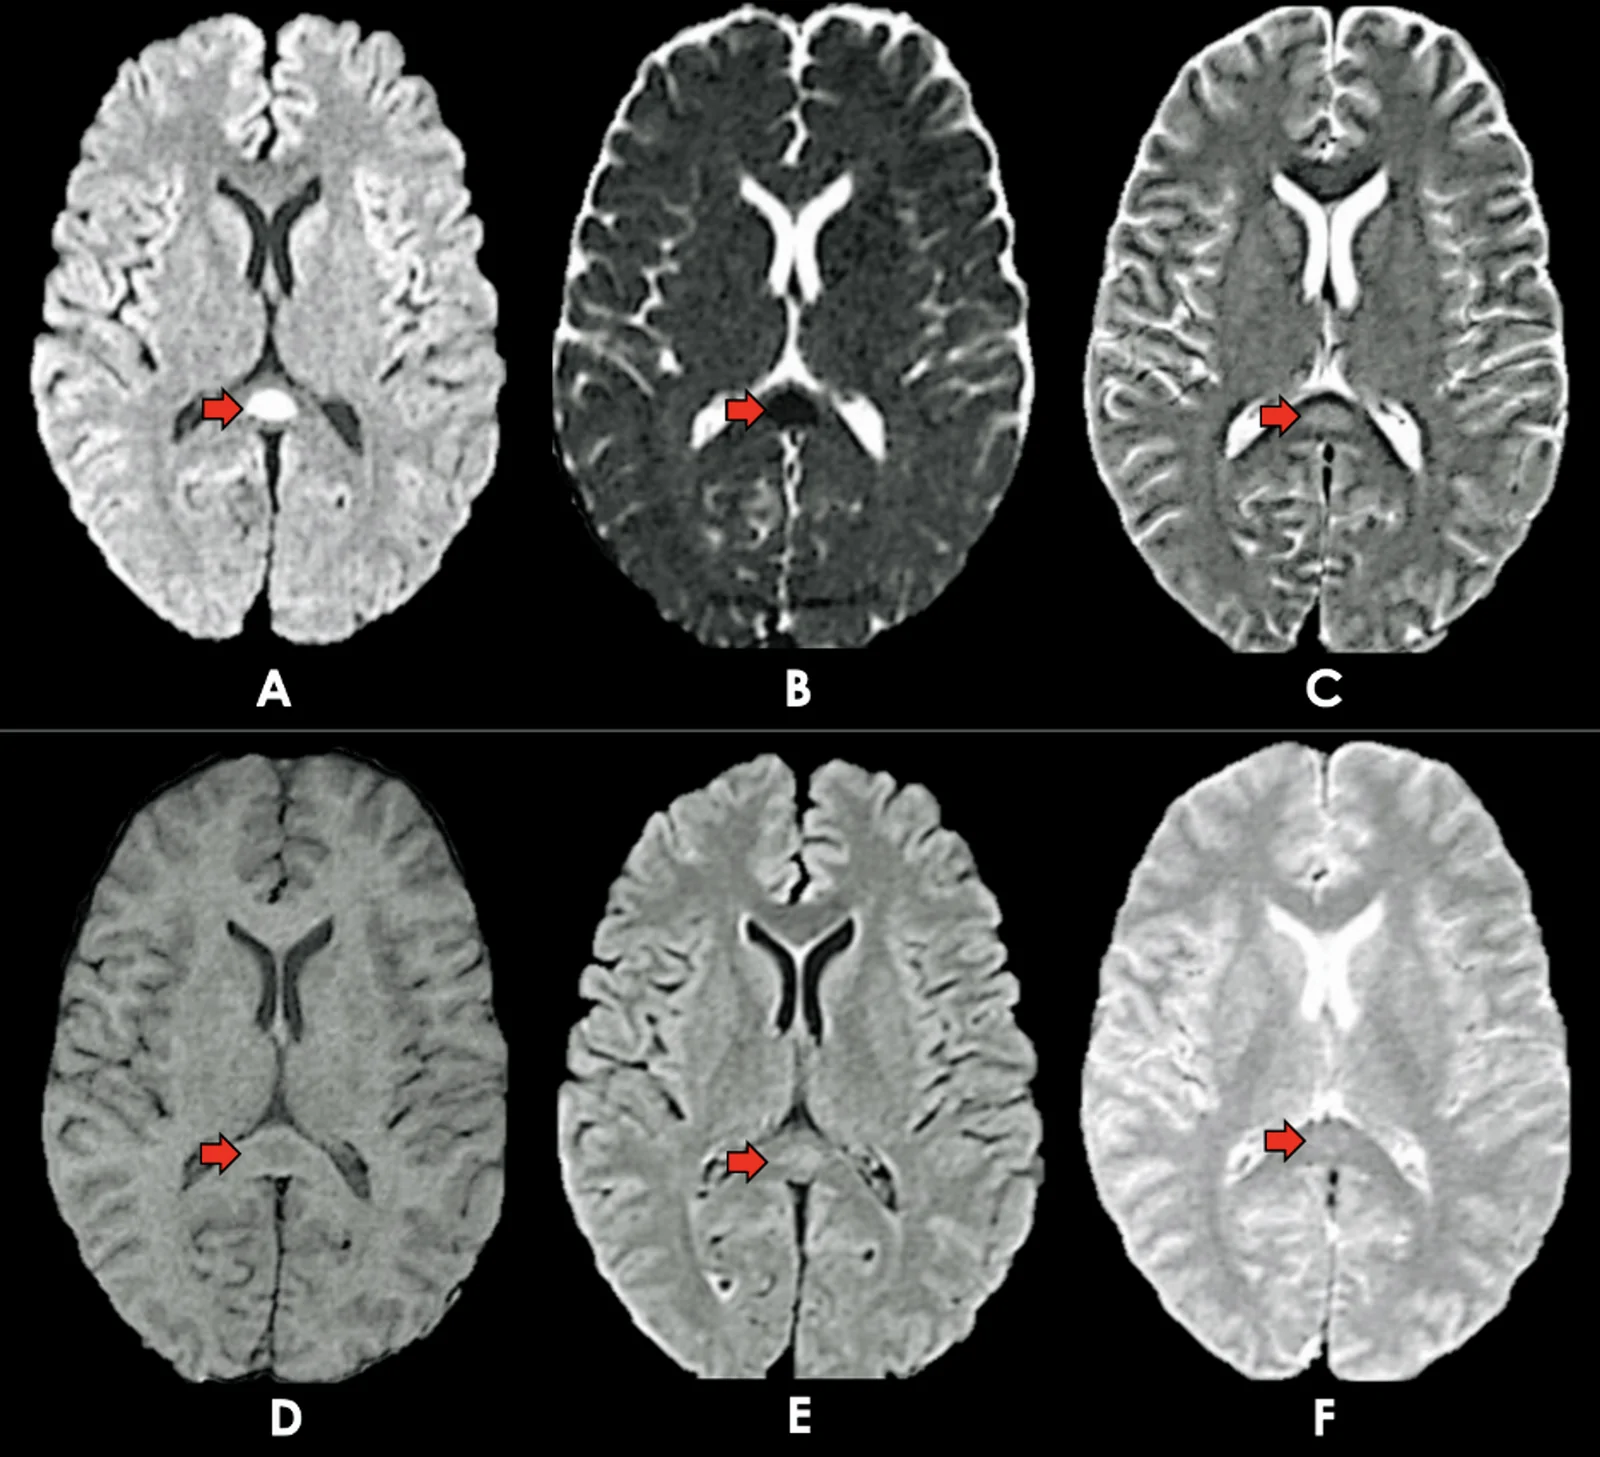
Initial cranial MRI demonstrating a focal cytotoxic lesion involving the splenium of the corpus callosum (arrow) (A) Diffusion-weighted imaging (DWI) demonstrates marked diffusion restriction. (B) Corresponding apparent diffusion coefficient (ADC) map shows low signal intensity, consistent with cytotoxic edema. (C) Axial T2-weighted image demonstrates subtle hyperintensity within the splenium. (D) Axial T1-weighted image demonstrates mild hypointensity within the splenium. (E) Axial fluid-attenuated inversion recovery (FLAIR) image demonstrates subtle hyperintensity within the splenium. (F) Gradient-recalled echo (GRE) sequence demonstrates no evidence of hemorrhage. MRI = magnetic resonance imaging
